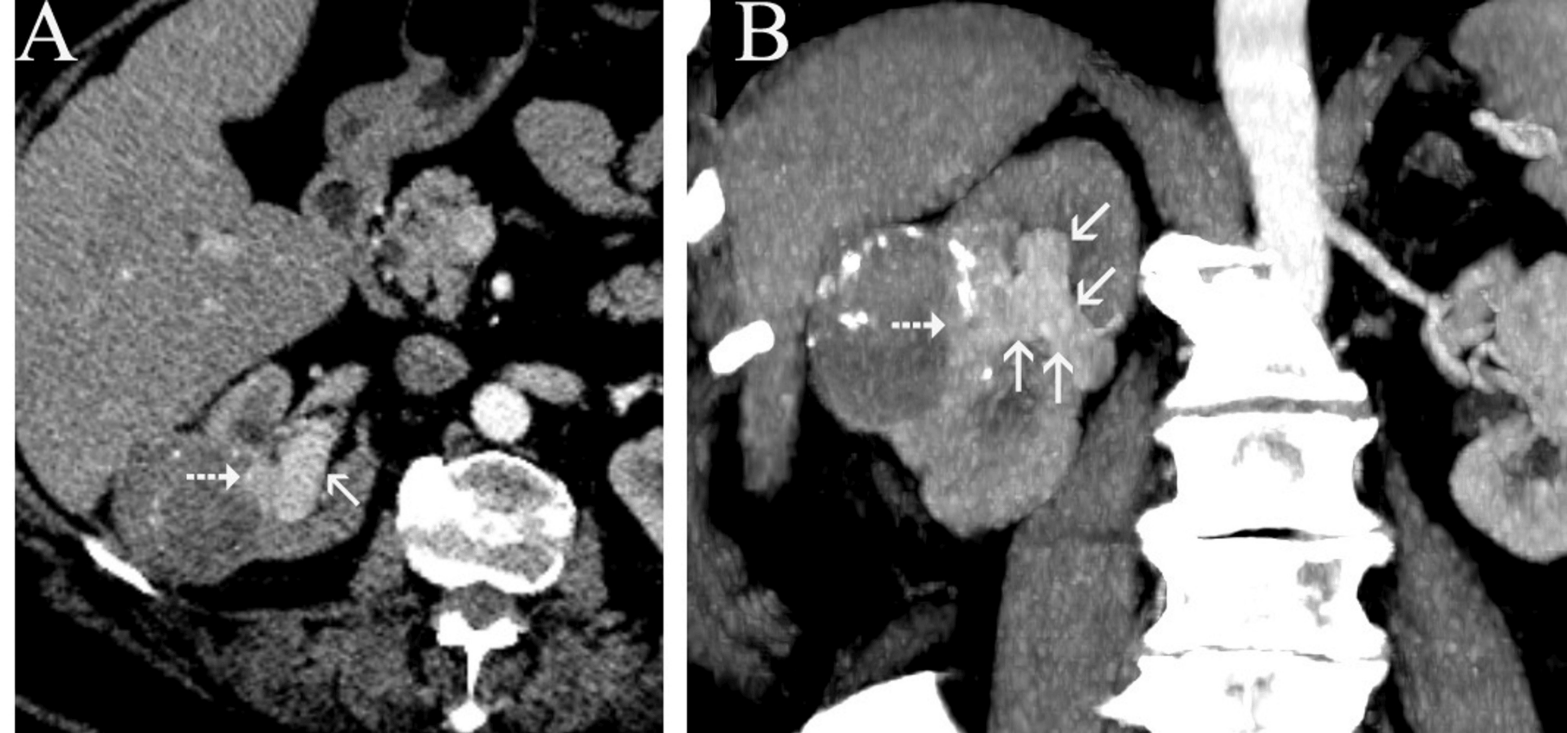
Contrast-enhanced, axial CT image (A) and coronal-oblique maximum intensity reconstruction (B), 10 weeks post ablation. Minimal residual enhancing tumor (dotted arrows in A and B), and marked involvement and dilatation of the pelvicalyceal system by enhancing tumor (arrows in A and B). Note the continuity and the similar enhancement between the residual and the newly appearing intrapelvicalyceal tumor.
